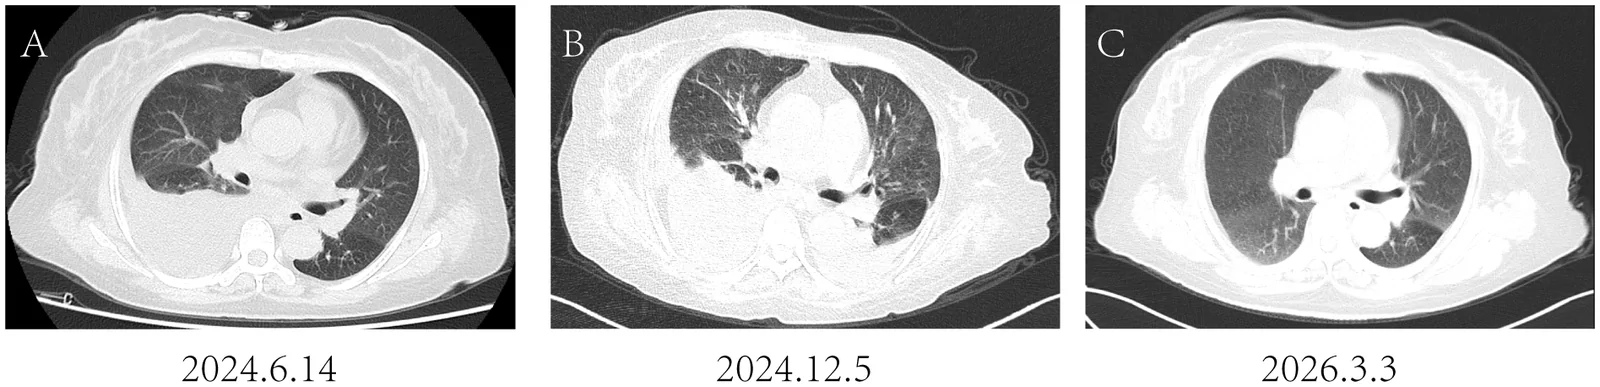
Serial chest CT images of a patient: (A) Before treatment; (B) After glucocorticoid and immunosuppressive therapy; (C) After PCSK9 inhibitor therapy CT, computed tomography, PCSK9, proprotein convertase subtilisin/kexin type 9.
